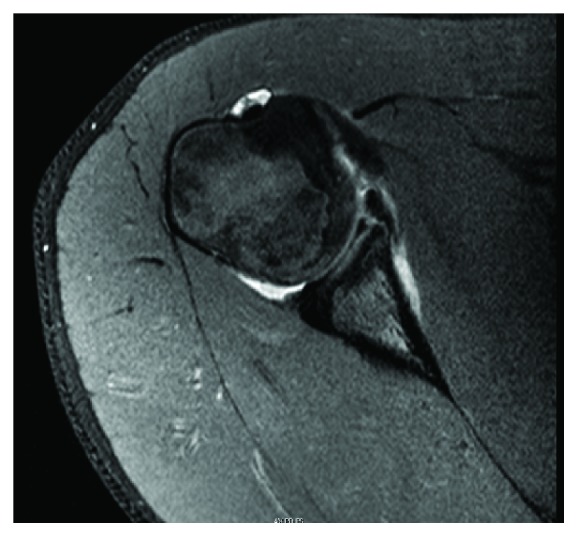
Preoperative MRI that was initially read as a Buford complex, but after failed conservative management, the lesion was reevaluated as an anterior inferior labral tear.
